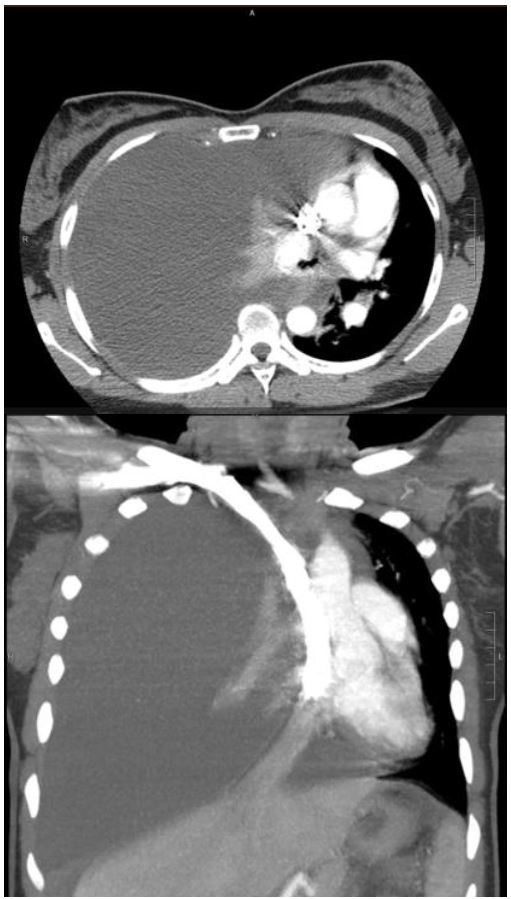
Axial (A) and coronal (B) computed tomography angiogram images of the chest showing a large right pleural effusion (arrows) and near-complete atelectasis of the right lung (arrow heads) with leftward mediastinal shift.
